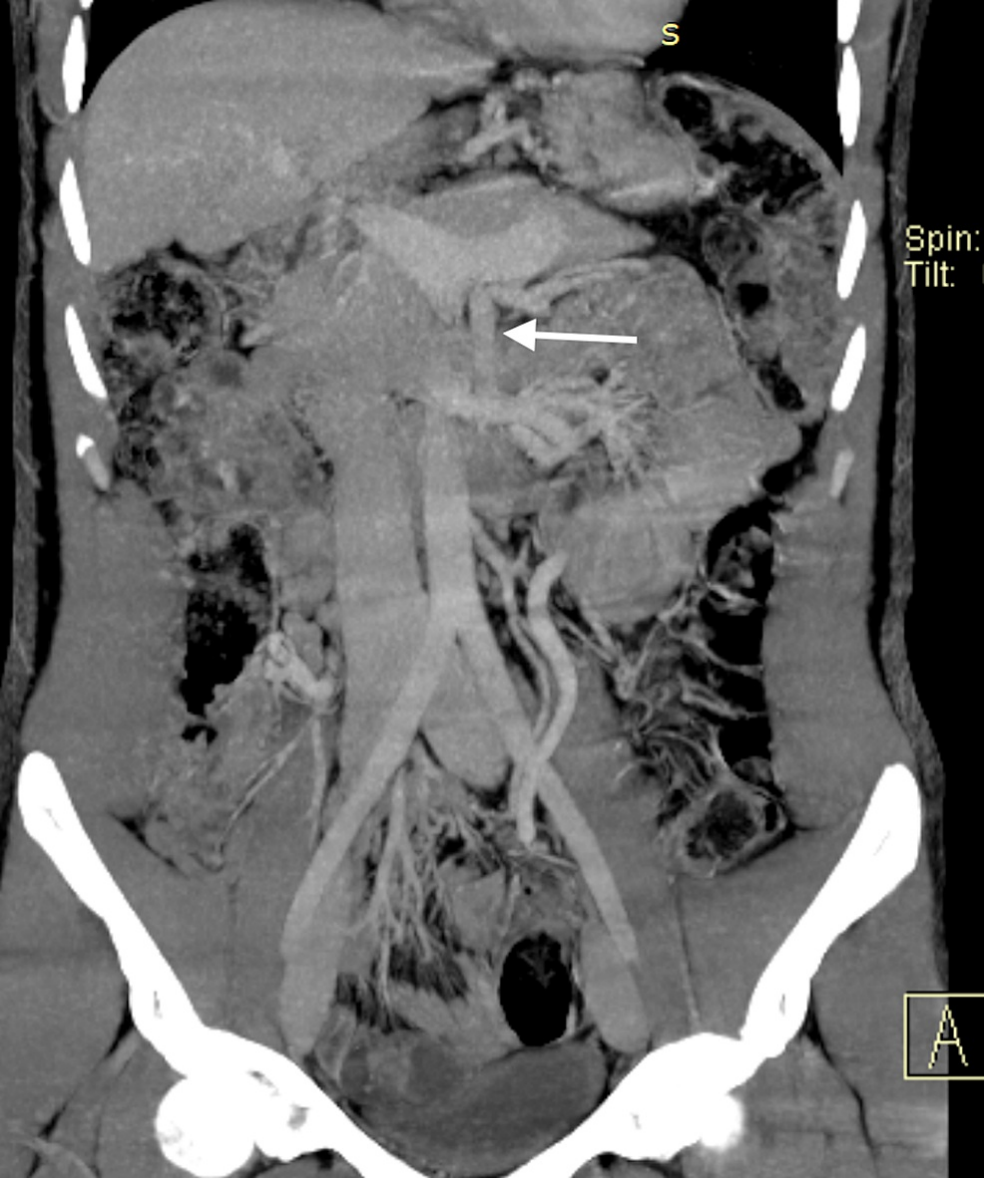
Coronal section of abdominal CT after a splenorenal shunt. Coronal CT scan showing a patent splenorenal shunt in place (arrow).
